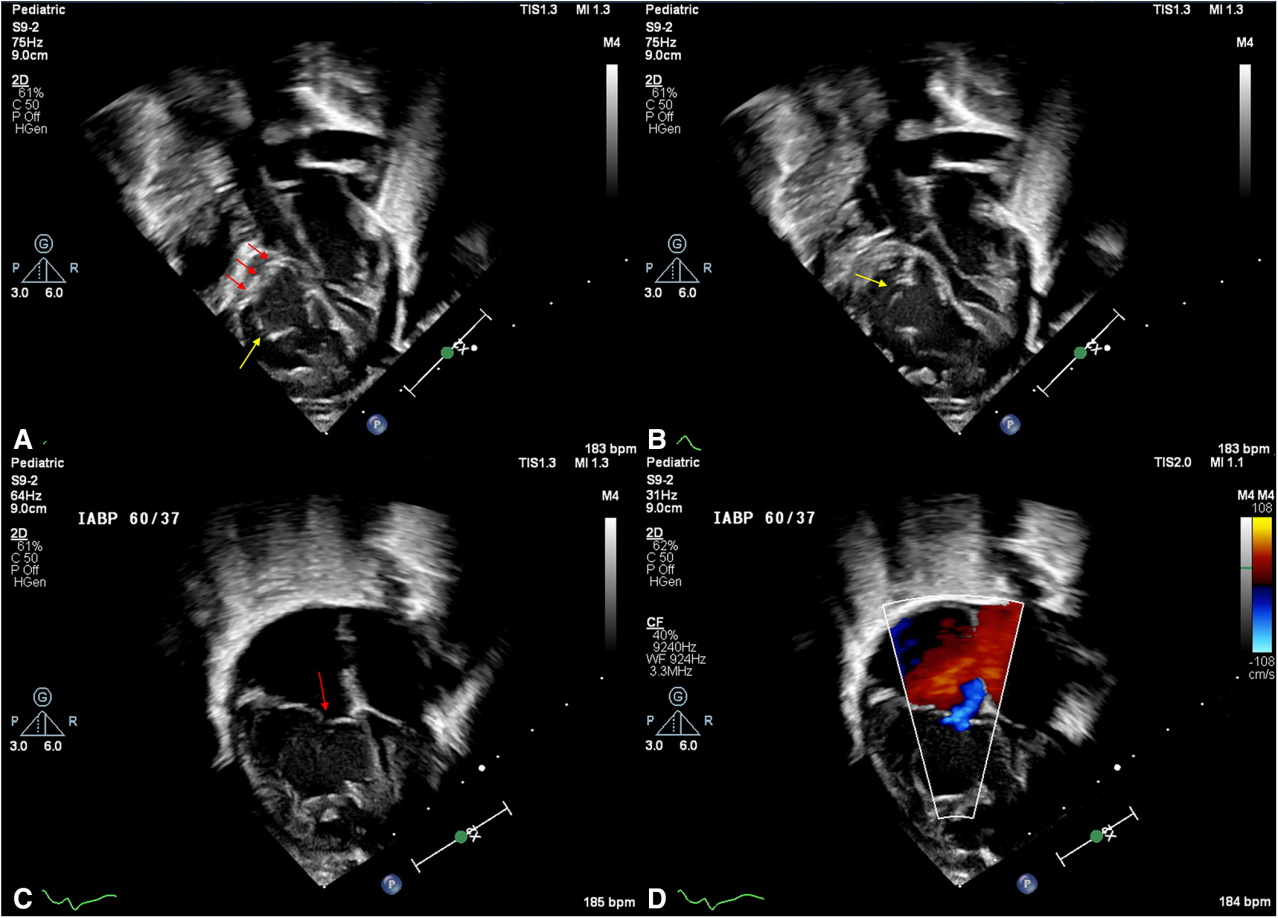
Tricuspid valve malformations in HLHS. (A) Subcostal view of the tricuspid valve in early-mid diastole. The anterior leaflet free margin is bright and thickened (red arrows), and a deep cleft is noted at the antero-inferior commissure. (B) Subcostal view in late diastole. An additional cleft and nodules are noted on the anterior leaflet (yellow arrow). (C) Apical 4 chamber view in early systole. Note the poor coaptation between anterior and septal leaflets (red arrow) and, (D) colour Doppler interrogation in the same view showing mild tricuspid regurgitation.
